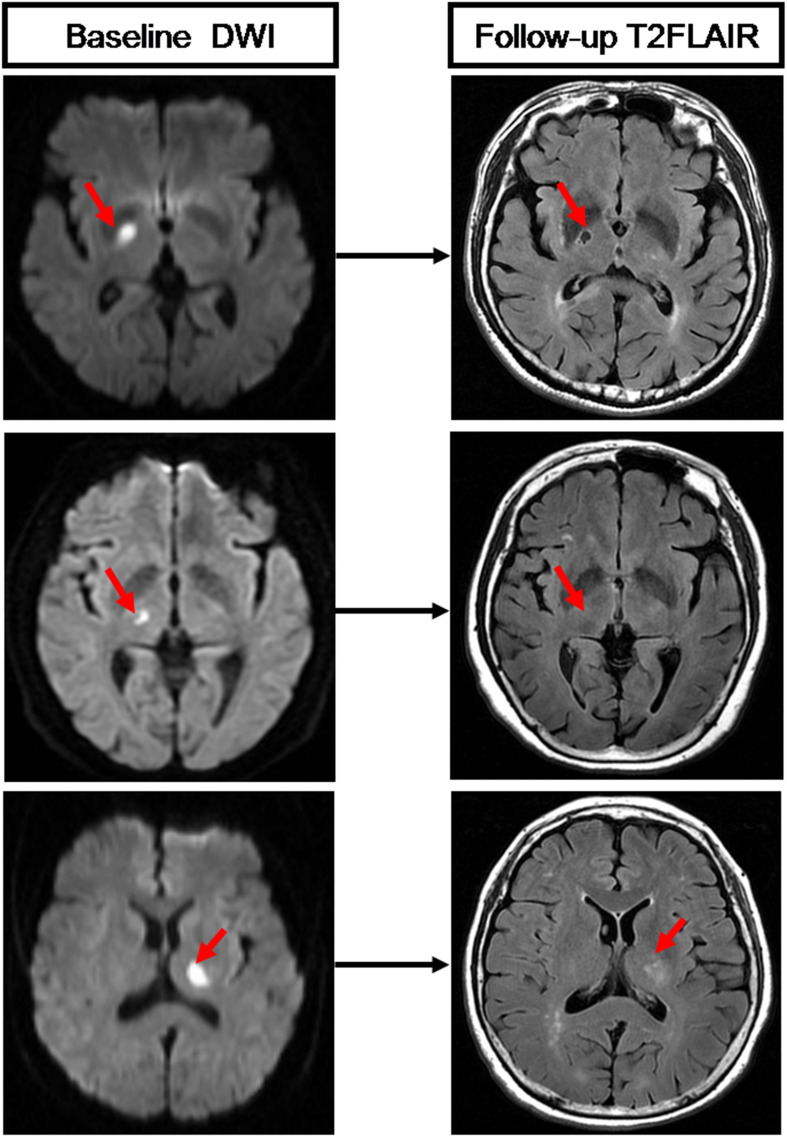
Three RSSIs show different evolution patterns. The top row shows an RSSI (red arrow) has evolved into a lacune on follow-up T2 FLAIR. The medium row shows an RSSI (red arrow) has disappeared on follow-up T2 FLAIR. The bottom row shows an RSSI (red arrow) that remained as white matter hyperintensities on follow-up T2 FLAIR.
